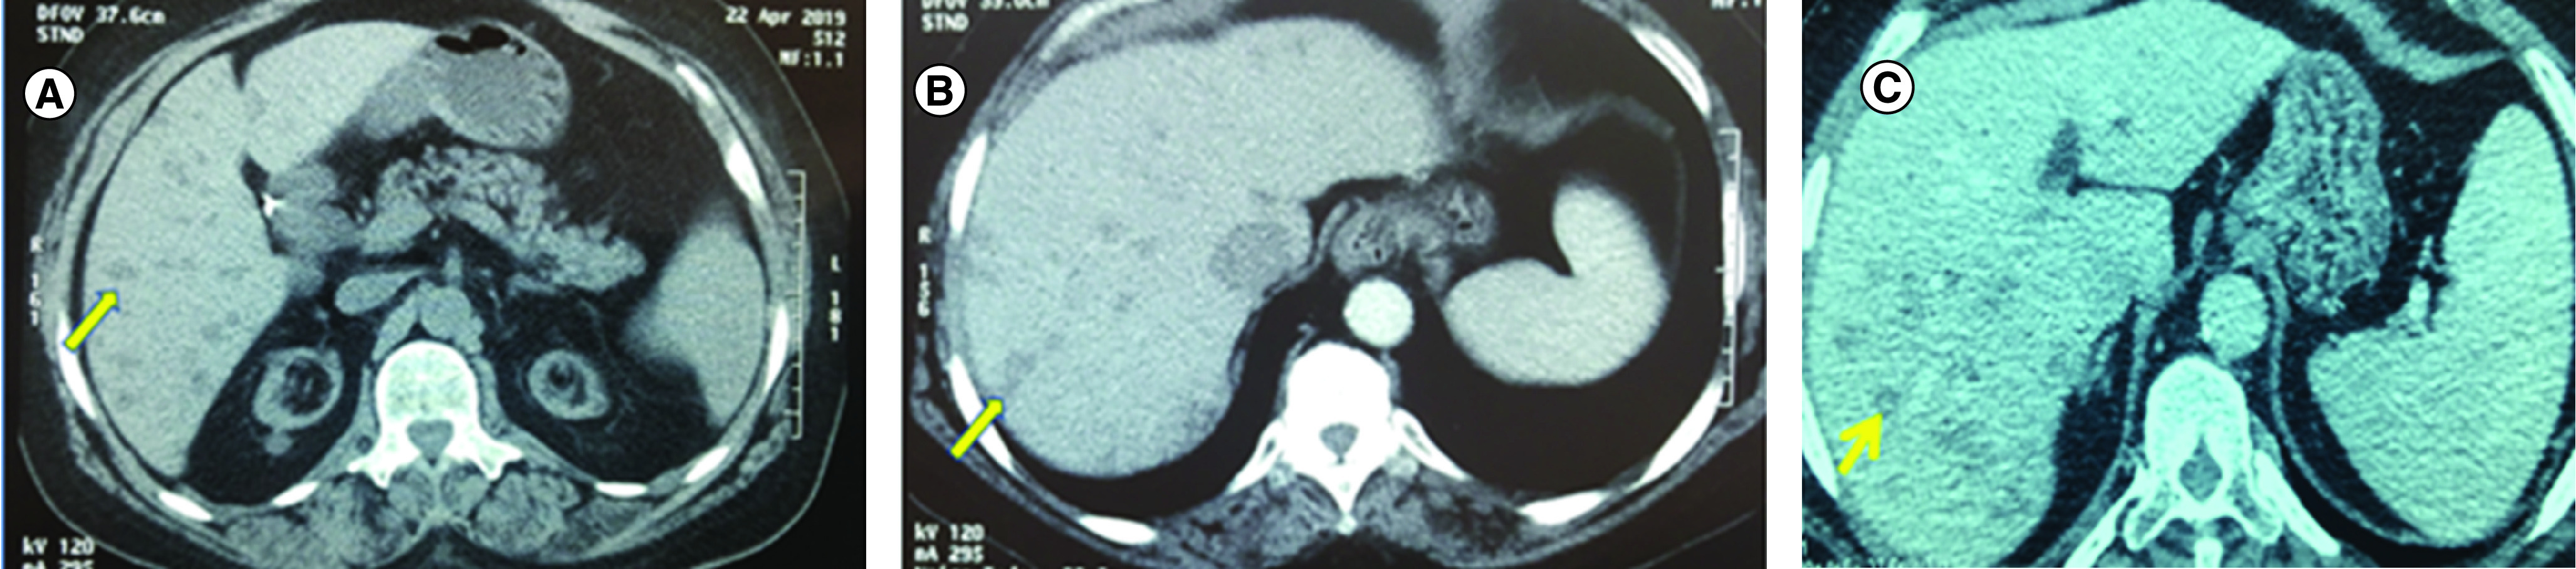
CT scan images in different phases. (A) Non-contrast enhanced CT scan showing multiple hypodense lesions. (B) Same findings on contrast enhanced CT scan in the arterial phase. (C) Same findings on contrast enhanced CT scan in the portal phase.
